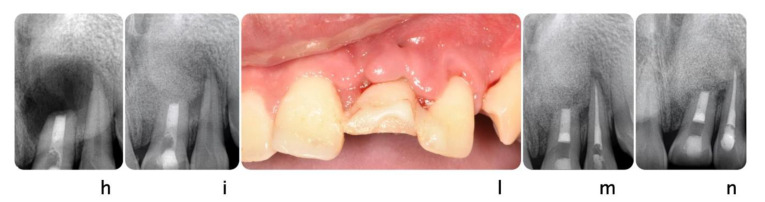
Clinical scenario of a 17 years-old adolescent hit by a baseball ball in the anterior maxillary region. The boy presented to the Periodontal Department at Tufts University, Boston USA with crown fracture of left central incisor (#9) (a,b). Peri-apical radiograph show apical radiolucency, sign of necrosis. After the diagnosis, CaOH_2_ was applied. The root canal definitive treatment was completed but after 2 months the patient still presented with a fistula, that was tracked via a gutta-percha point. A CBCT scan was performed in order to diagnose the extent of the peri-apical lesion (c in sequence). The extent of the lesion did not suggest an endodontic therapy revision. Exploratory surgery was performed in order to rule out tooth fracture (d). The apex was resected in order to access the palatal aspect of the tooth. A PA radiograph was taken in order to verify correct apex resection and endodontic retrograde seal (e). Due to active patient skeletal growth, a decision was made to enucleate the endodontic cyst and treat the cavity with bone grafting material, in order to preserve the site for future implant placement (f,g). PA radiograph comparison before and after grafting placement (h,i). The patient was followed up for 2 months, and a fistula was identified apical to #9 (l). Tooth #10 was diagnosed as necrotic. A root canal was performed (m) and the apical radiolucency and fistula were resolved at 1 month follow up (n).
